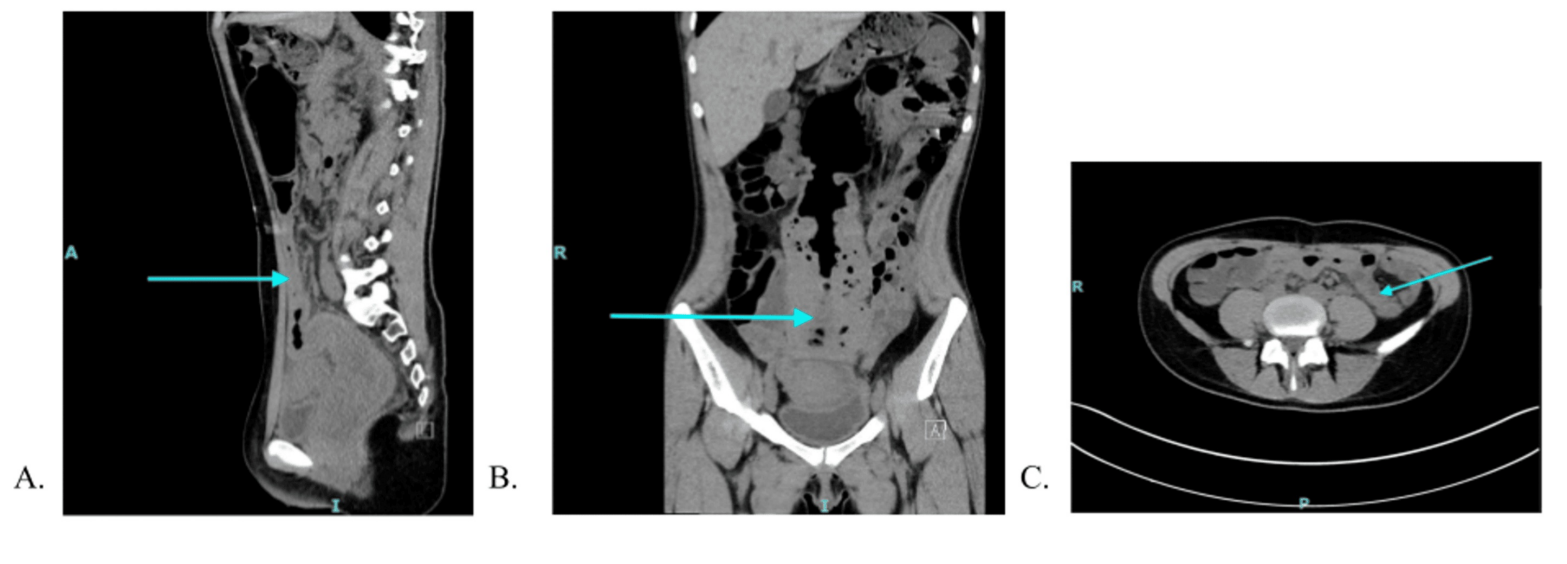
Repeat CT abdomen/pelvis with IV contrast on the day of discharge, demonstrating the colon cleared of stool A: Sagittal view. B: Coronal view. C: Transverse view. The blue arrow points at the formerly most distended portion of the colon post-medical and endoscopic management.
